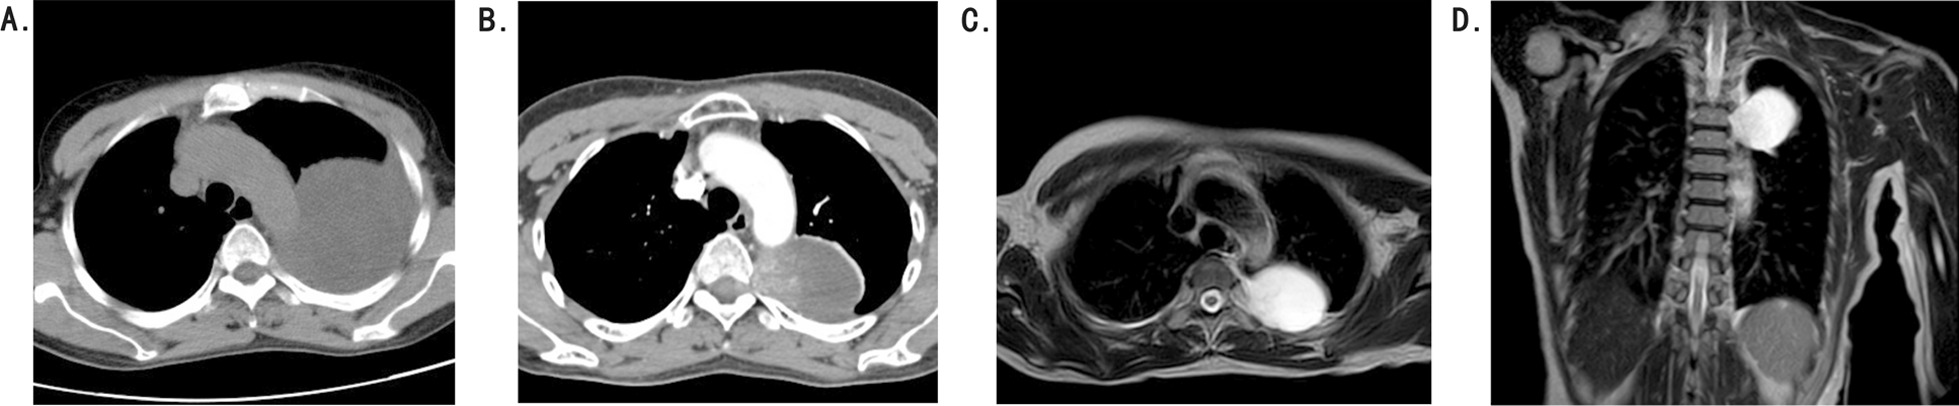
CT showing a large left-side pleural effusion (A). Enhanced CT showing a posterior mediastinal mass (B). T2-weighted contrast-enhanced sections showing heterogeneously enhanced (C), Axial and (D), coronal images
